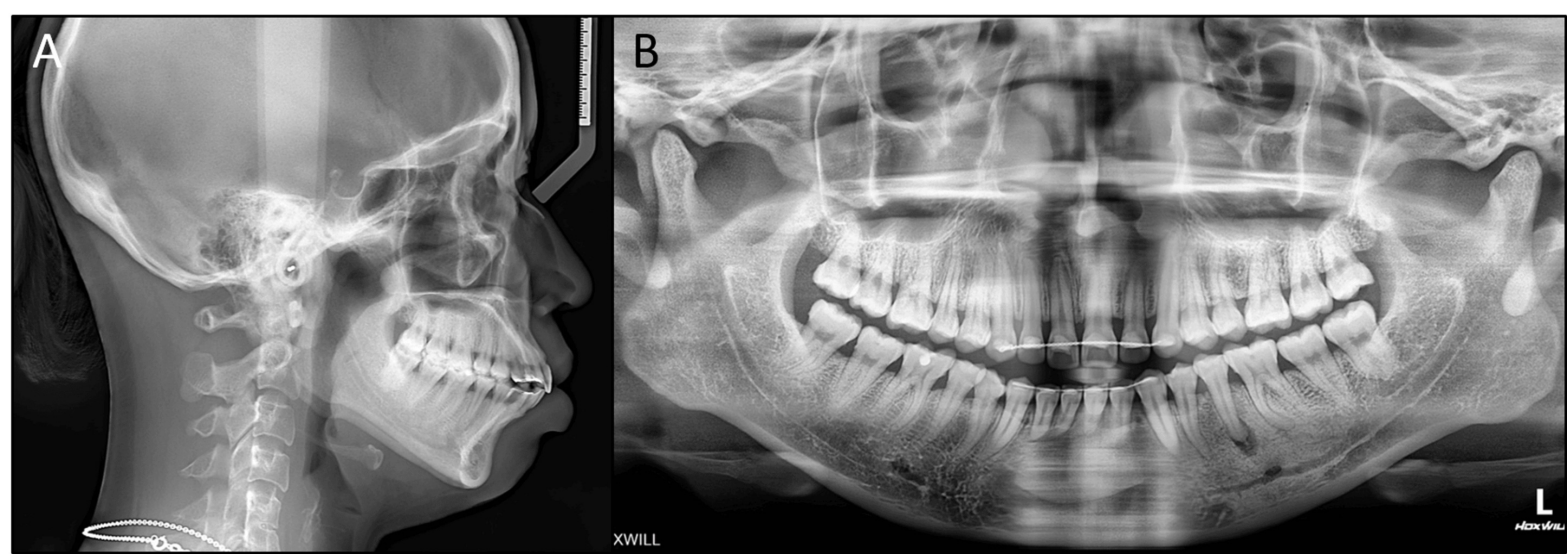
Pre-treatment radiographs. (A) Lateral cephalometric radiograph, (B) panoramic radiograph.
